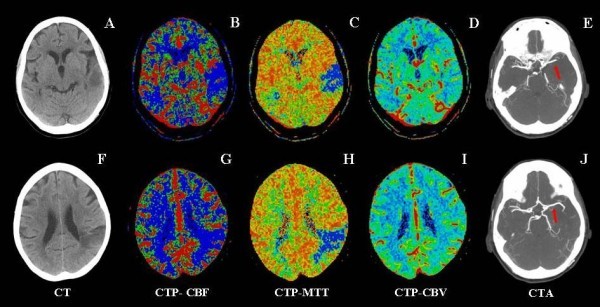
CT protocol performed at 7 days after stroke onset. *A and F*: unenhanced CT scans (ASPECTS levels) show the appearance of two areas of hypoattenuation in the left temporal and parietal cortex; *B-D and F-H*: CTP maps (ASPECTS levels) demonstrate the persistence of two areas of hypoperfusion in CBF and MTT maps and show the normalization of the CBV defect in the territory of the left middle cerebral artery; *E and J*: axial CTA MIP images shows the complete recanalization of the M1 segment of the left middle cerebral artery (*arrows*).
